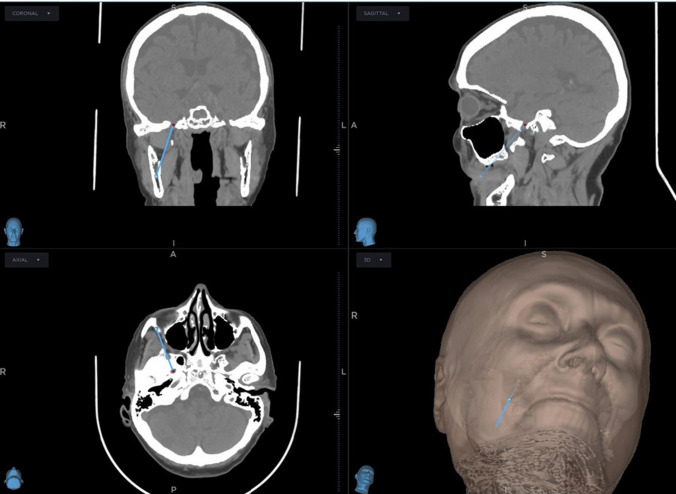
Preoperative neuronavigation planning for percutaneous balloon compression. Coronal, sagittal, and axial CT views demonstrate the planned needle trajectory (blue line) targeting the foramen ovale. A 3D surface reconstruction confirms the cutaneous entry point relative to facial landmarks, allowing trajectory optimization and avoidance of bony collision
